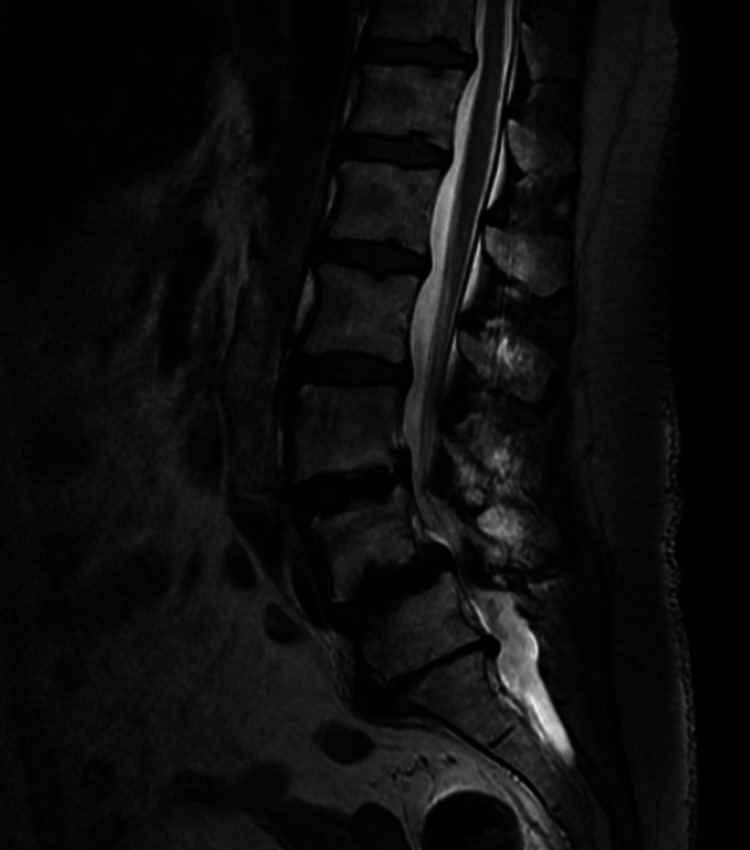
Preoperative lumbar MRI demonstrating L5-S1 disc bulge with disc collapse and modic changes at L5 and S1 loss of segmental lordosis secondary to ASD. MRI, magnetic resonance imaging; ASD, adjacent segment disease.
